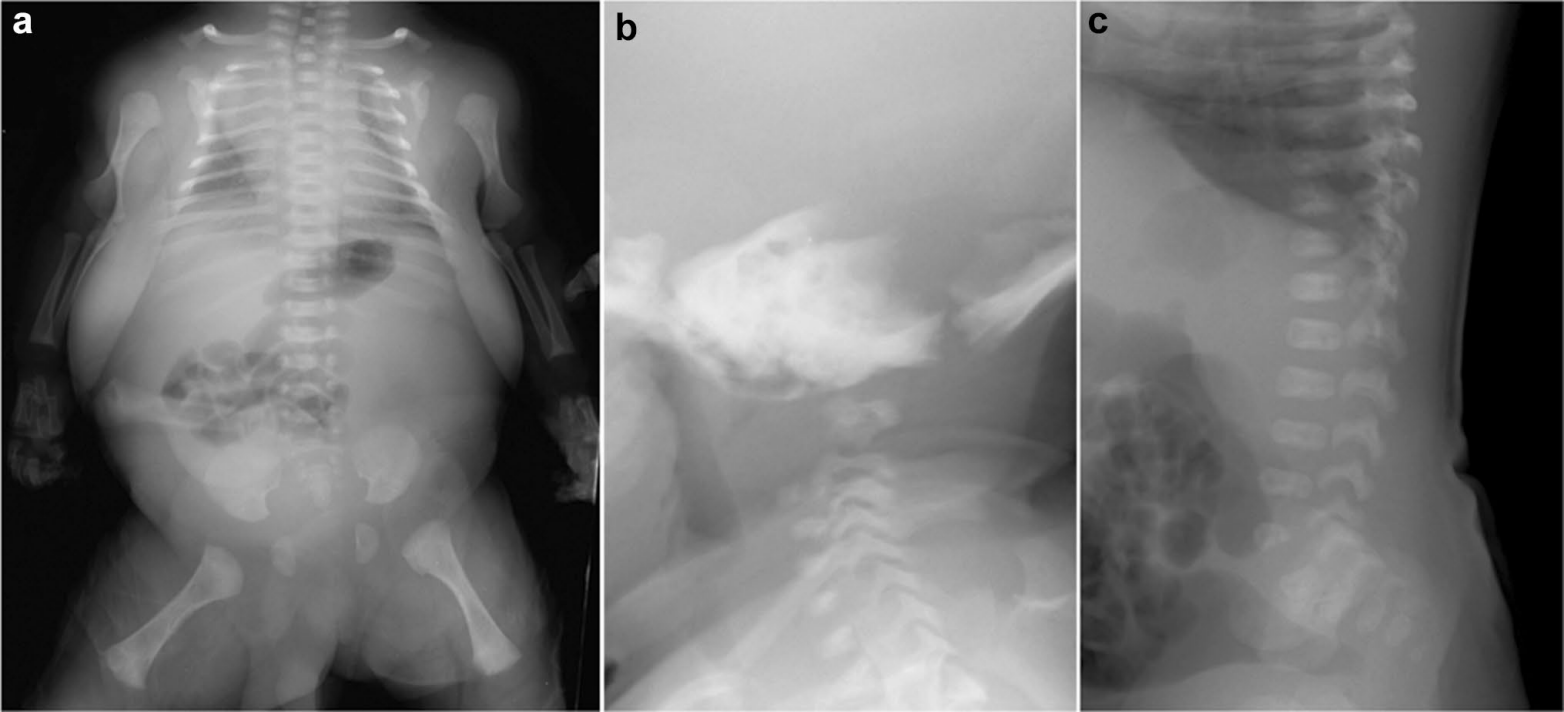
a–c A neonate with SEDC. Radiographs show a broad thorax and modest platyspondyly (flattening of the vertebral bodies) due to delayed vertebral ossification. The delayed ossification is prominent in the dorsal vertebral bodies, giving rise to pear-shaped vertebral bodies seen on the lateral view. The delayed ossification is more severe in the cervical spine and sacrum than in the thoracolumbar spine, creating anisospondyly (increased variability of the size of the vertebral bodies). The ilia are craniocaudally short, the pubic bones are absent, epiphyseal ossification of the distal femora is retarded (typically seen by 39 weeks of gestation), and the long bones show mild metaphyseal widening
